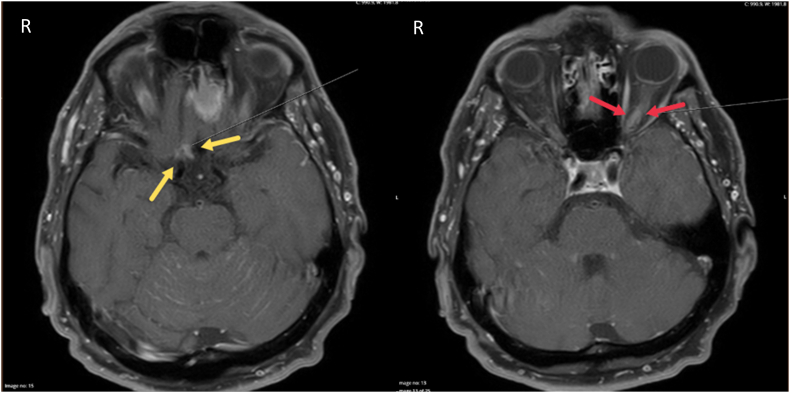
Magnetic resonance imaging (MRI) of the brain and orbits in a 73-year-old man with history of solitary extramedullary plasmacytoma (SEP), performed at presentation, 3 years after the diagnosis of SEP, shows enhancement in both the right (yellow arrows) and left (red arrows) optic nerves, suggesting the recurrence of the previously diagnosed SEP that had been treated with surgery and curative radiotherapy. (For interpretation of the references to colour in this figure legend, the reader is referred to the Web version of this article.)
